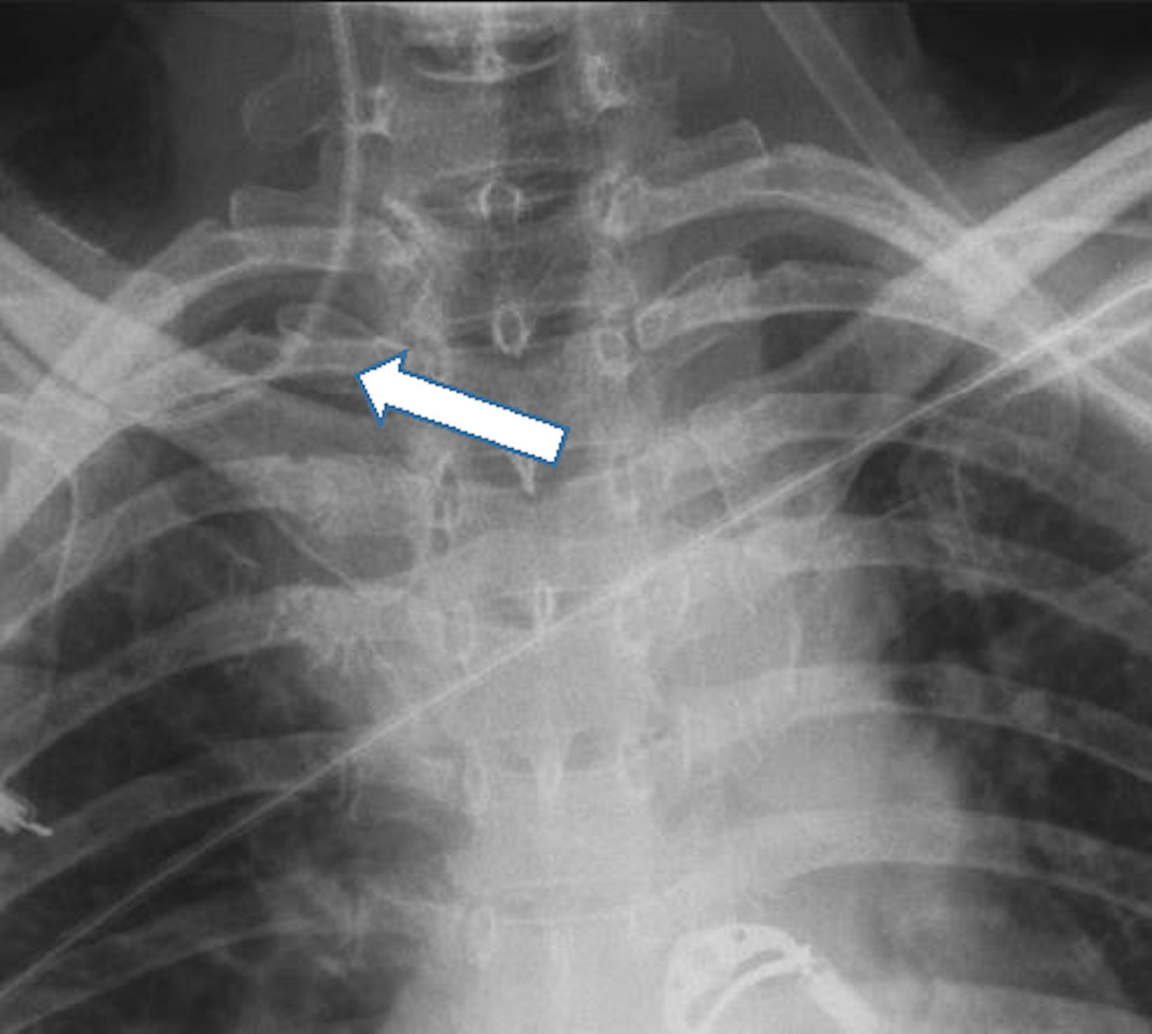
Portable chest radiograph showing a central line catheter placed on the right subclavian vein. The catheter migrated to the right internal jugular vein despite proper procedural technique. Mild resistance was experienced during the wire threading. The patient had history of chronic renal disease. A hemodialysis catheter had been placed in the right subclavian vein for several months and had been removed recently.
